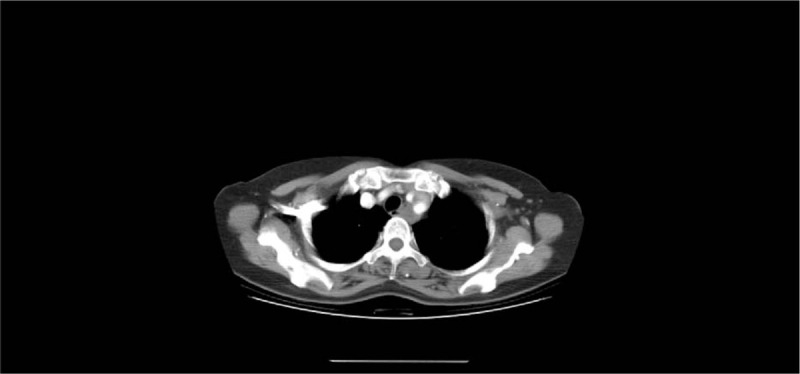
Contrast-enhanced computed tomography (CT) of the thoracic region revealed well-defined luminal protruding nodule at the upper-esophagus.
